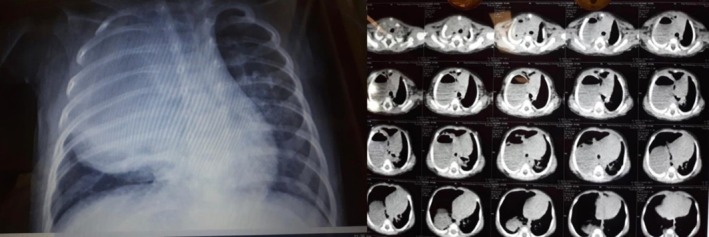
Chest x‐ray (Left) and chest CT scan (Right) of patient showing abscess in right upper lobe (before surgical operation).
